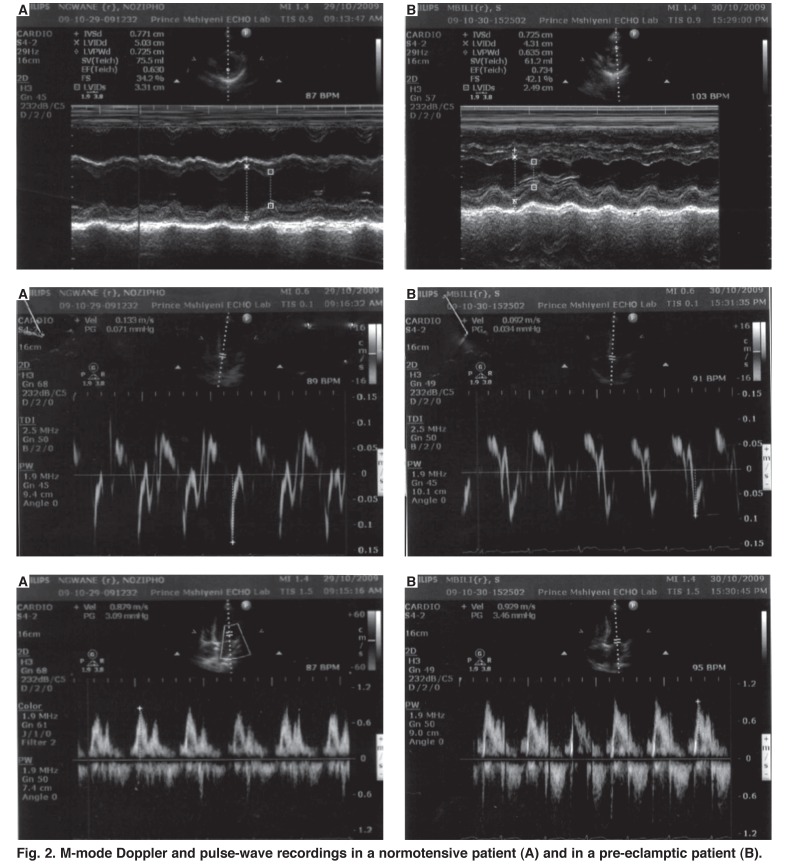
M-mode Doppler and pulse-wave recordings in a normotensive patient (A) and in a pre-eclamptic patient (B).
